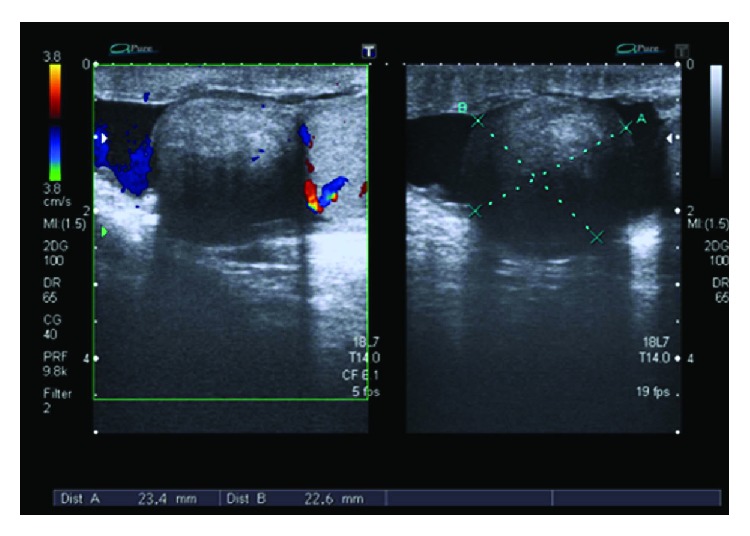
Gray scale and Doppler ultrasound scans showing well-circumscribed lesion separated from the testis and relatively hypoechoic compared to testicular parenchyma with no significant vascularity on color Doppler.
